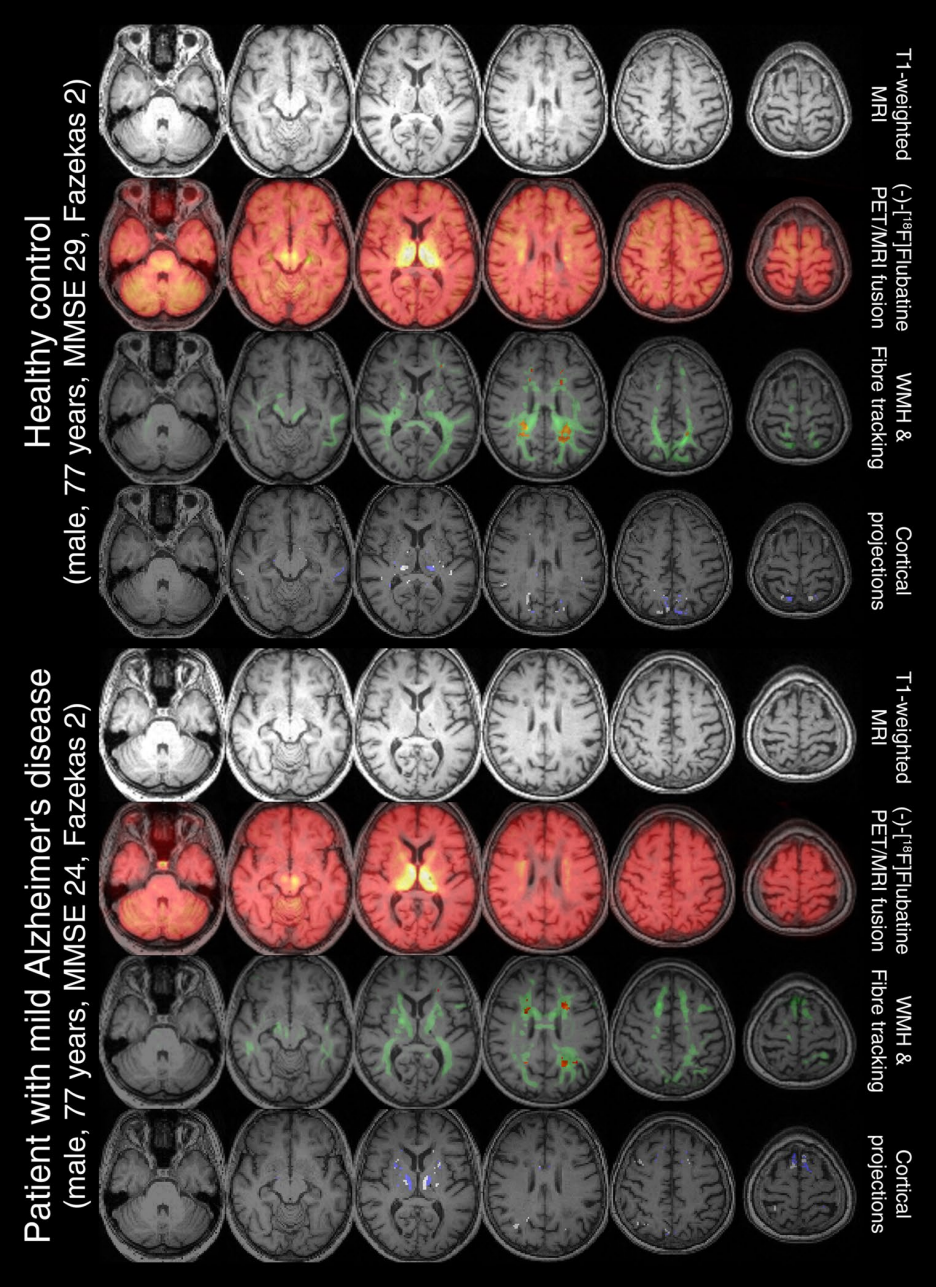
Two cases with a Fazekas score of 2 are presented showcasing its different imaging and processing modalities. For each case, the first row displays T1-weighted MR images, while the second row introduces a fused view of (-)-[^18^F]Flubatine PET images. The third row overlays the identified white matter hyperintensities (WMH) in red along with he corresponding fiber tracts in green, as determined by fiber tracking. The fourth row highlights the WMH-connected gray matter (blue) and their unaffected contralateral counterparts (white), which were used to extract PET-based distribution volumes. MMSE: mini-mental state examination
